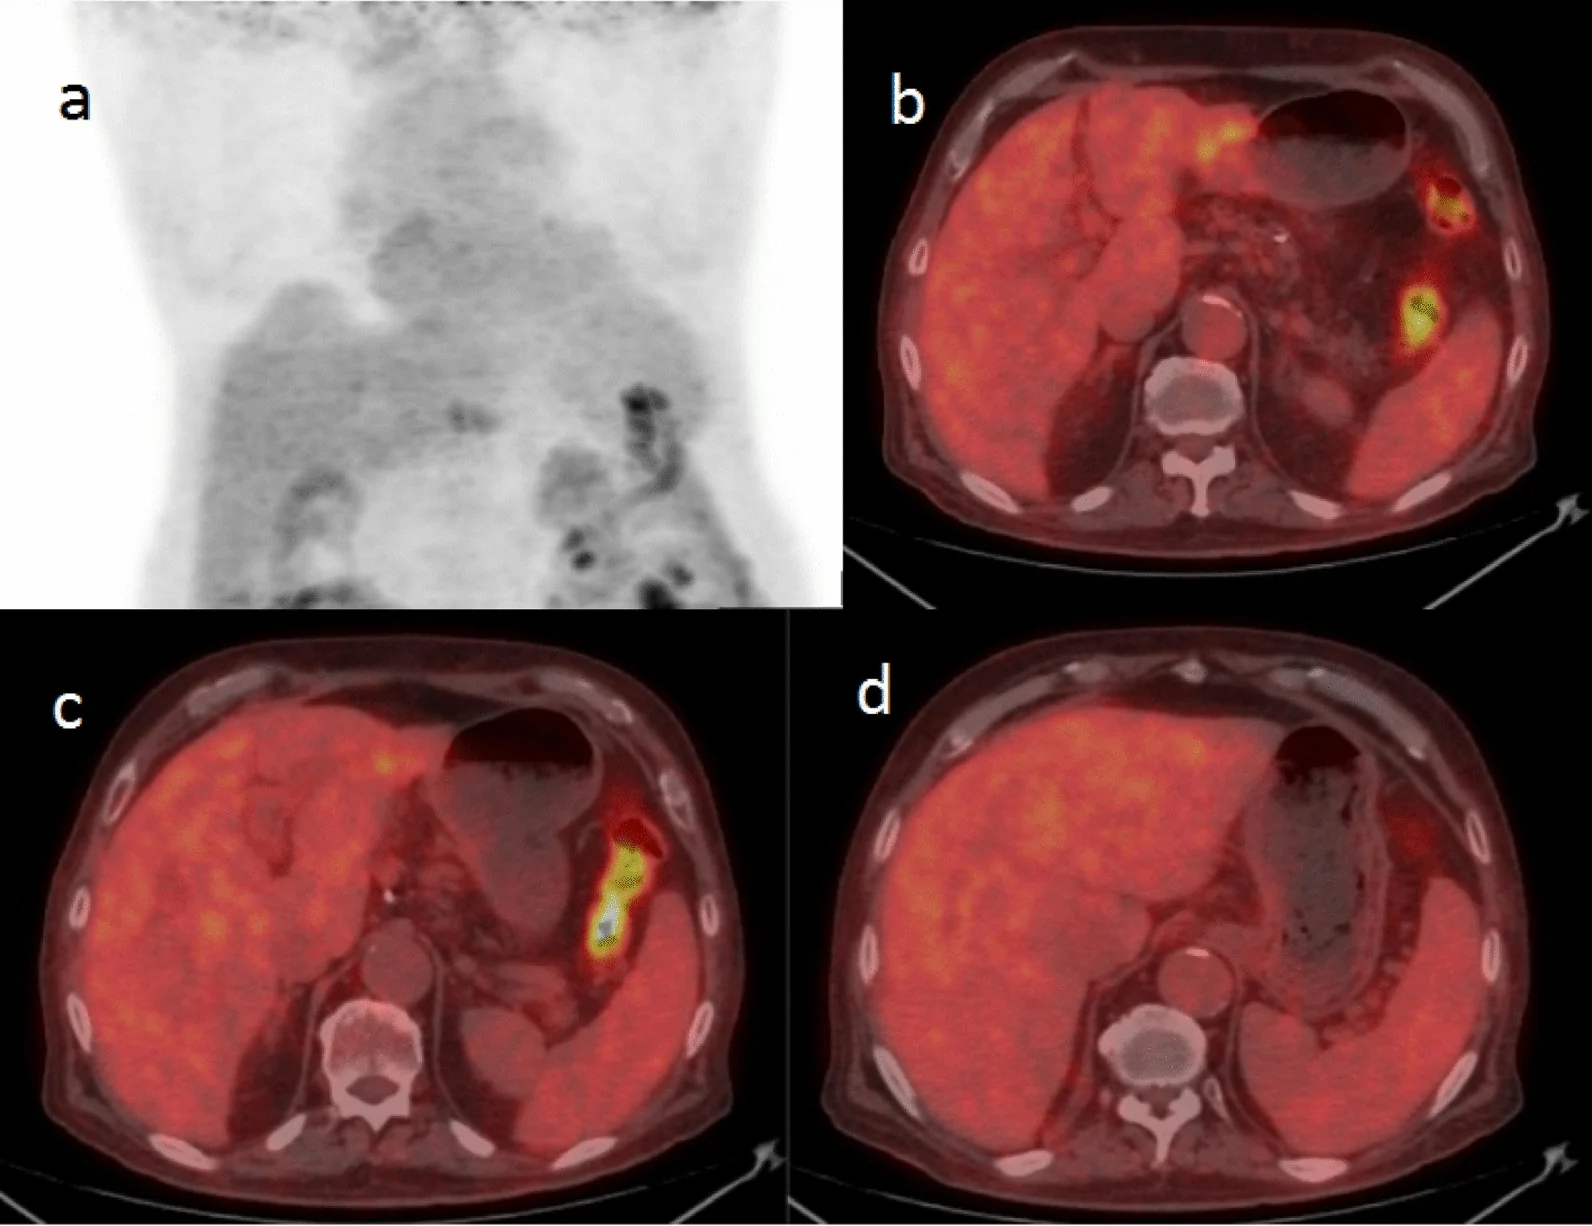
Positron emission tomography–computed tomography scan at 1-year follow-up demonstrating complete metabolic response
